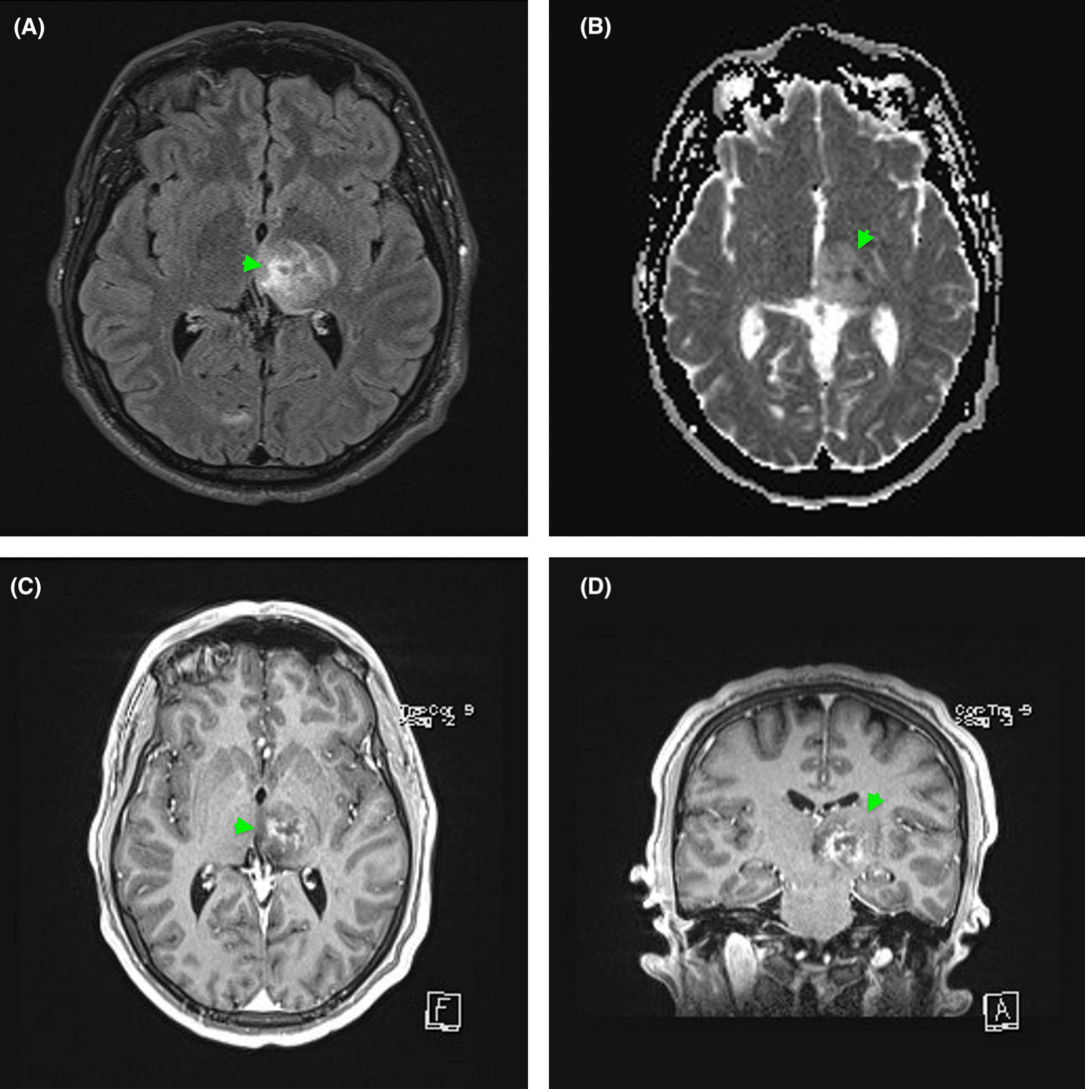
(A) Demonstrates an axial fluid-attenuated inversion recovery (FLAIR) post-contrast sequence. (B) Demonstrates an axial apparent diffusion coefficient (ADC) map sequence. (C) Demonstrates an axial T1-weighted post-contrast sequence. (D) Demonstrates a coronal T1-weighted post-contrast sequence. The brain MRI demonstrates a left-sided thalamic irregular ring-enhancing space-occupying lesion (SOL) associated with diffusion restriction. Images demonstrate the presence of a lesion surrounded by peri-focal oedema with a small, predominantly linear abnormal signal in the right inferior midbrain.
